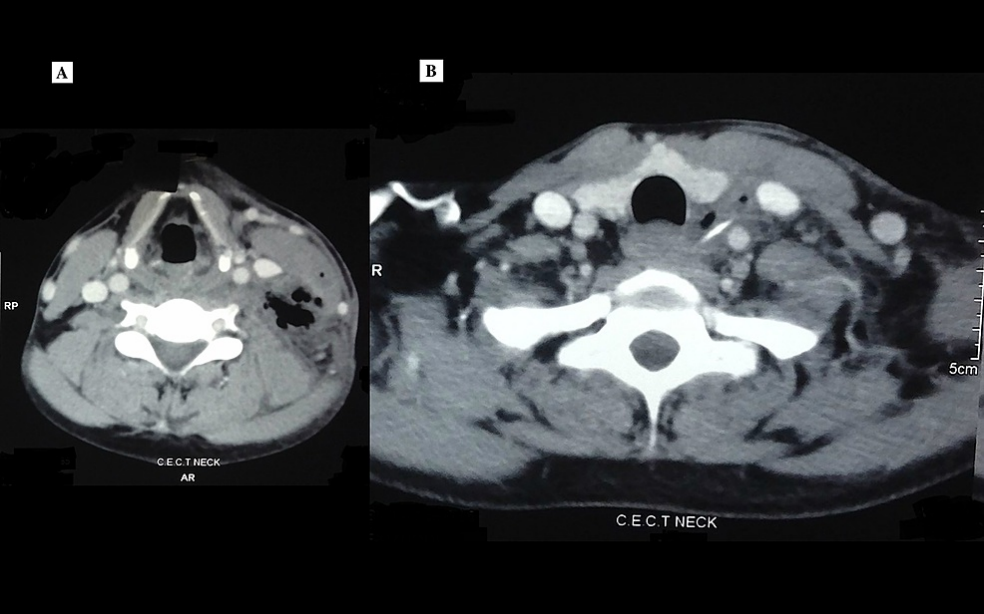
(A) CT showing abscess in the left parapharyngeal space. (B) CT showing the foreign body.
